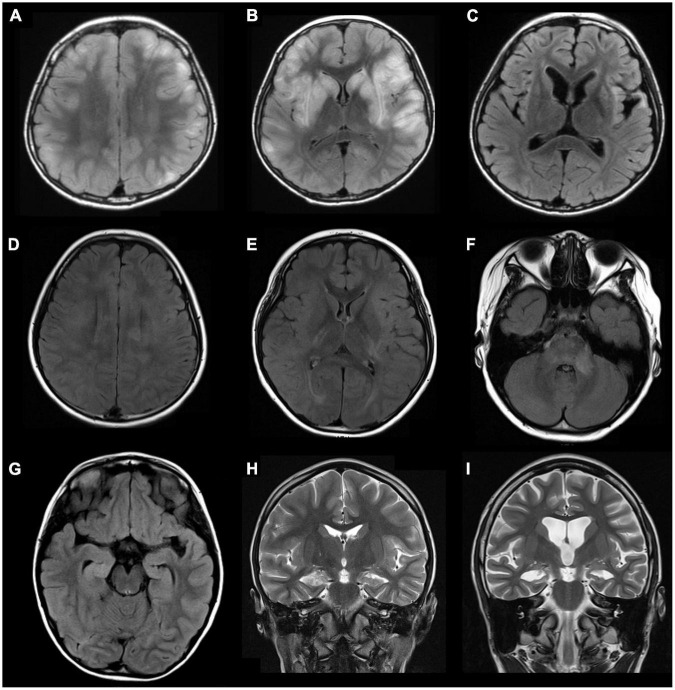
Examples of abnormal brain magnetic resonance imaging (MRI) findings in three patients with pediatric encephalitis/encephalopathy with anti-GAD antibody. Patient 1: A 10-year-old boy with clinical diagnosis of encephalitis with preceding influenza B infection 6 days ago. (A,B) Axial fluid-attenuated inversion recovery (FLAIR) sequences show multiple increased signals in the basal ganglia and cortical/subcortical regions of bilateral cerebral hemispheres (frontal, parietal, temporal lobes). (C) MRI follow-up at 9 months show progressive atrophy with stationary hyperintensities in bilateral putamen, bilateral insular regions, and left temporal tip. Patient 2: A 9-year-old girl with clinical diagnosis of rhombencephalitis with preceding HSV-1 infection 6 days ago. (D–F) Axial FLAIR sequences demonstrate multiple increased signals in the corpus callosum, bilateral insular regions, bilateral posterior capsules, and multiple cortical areas and white matter of bilateral hemispheres (all lobes), the brainstem, and left middle cerebellar peduncles. Patient 3: A 6-year-old girl with clinical diagnosis of febrile infection-related epilepsy syndrome (FIRES) without detected pathogen. (G) An axial FLAIR sequence and (H) a coronal T2 sequence show increased signals in right hippocampus and mesial temporal lobe. (I) Progressive cortical atrophy and right hippocampal atrophy were noted at the 30-month follow-up. GAD, glutamic acid decarboxylase.
